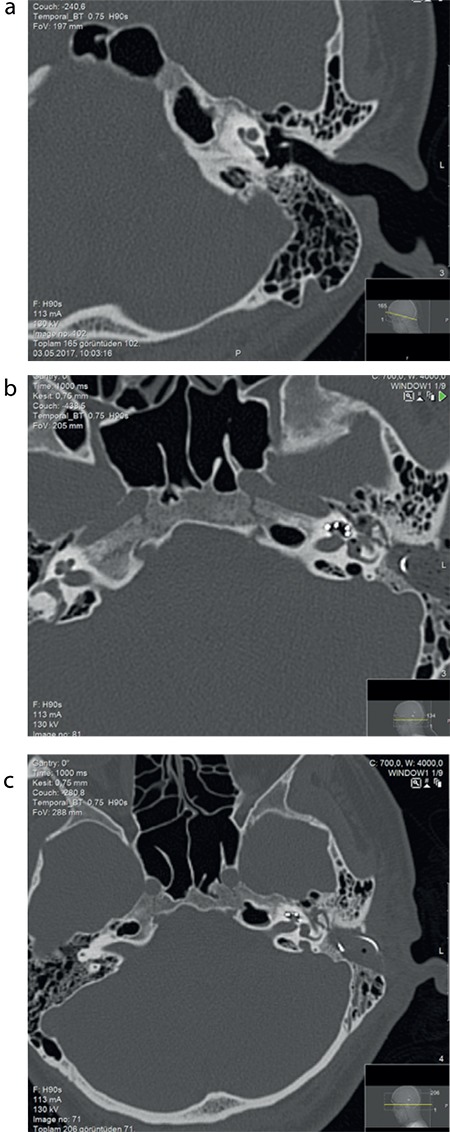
a-c. Computed tomography scan showing normal middle and inner ear structures with well pneumatized mastoid cells in the left ear (a), second computed tomography scan demonstrating the cochlear implant electrode in the cochlea in the early postoperative period. An air shadow was found in the inner ear, including cochlea, vestibule, and semicircular canals. This condition is defined as acute pneumolabyrinth (b), third control computed tomogrphy scan performed 6 days after the operation. The amount of air in the cochlea and vestibule in the control scan was lower than that in the second computed tomography scan (c).
